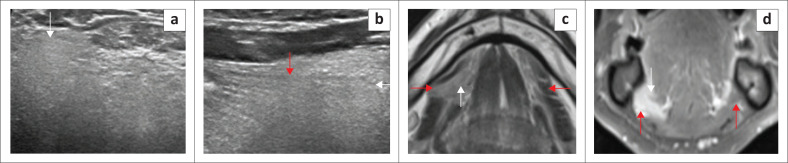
Mylohyoid Boutonniere in an adult patient who presented with a 2-year history of a fluctuating right submandibular neck lump. Ultrasound (a, b) demonstrate the enlarged inferiorly herniated right sublingual gland (white arrow) through the mylohyoid defect (red arrow). Subsequent MRI for ongoing concerns confirms the findings with a mylohyoid defect (red arrow) and herniated sublingual gland (white arrow) on the T2W axial image (c) and T1 contrast-enhanced fat-suppressed coronal image (d).
